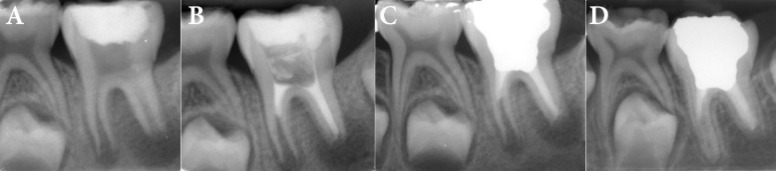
A)* Permanent immature first molar, with chronic apical periodontitis, went under emergency treatment; *B)* Mesial canals treated by revascularization protocol; distal canal was treated using apical plug technique; *C)* Tooth was permanently restored with amalgam; *D)* two-year follow-up radiography: resolved periradicular lesions and continued development of mesial root
